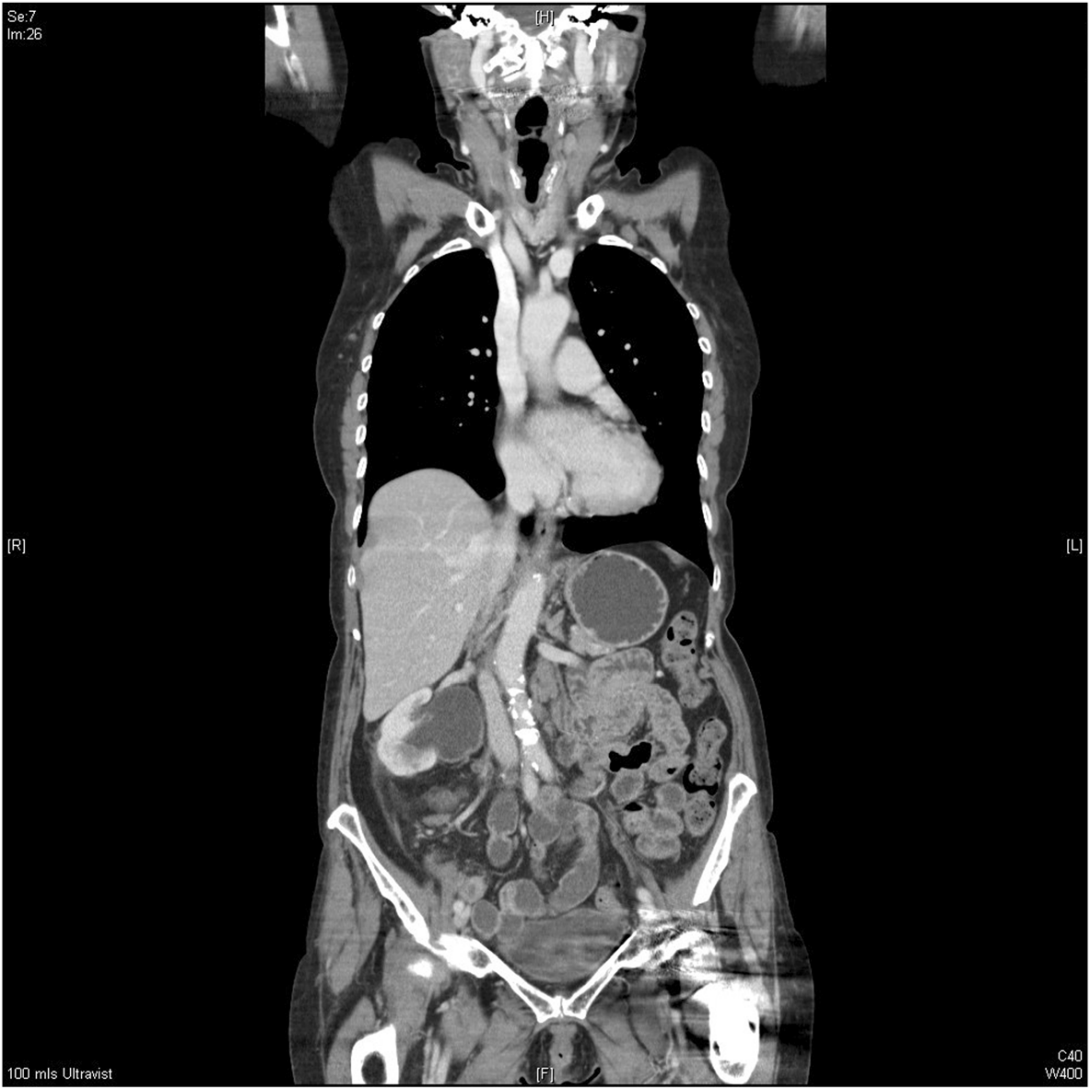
Computed tomography scan with persisting right PUJO and minor lymphadenopathy.
